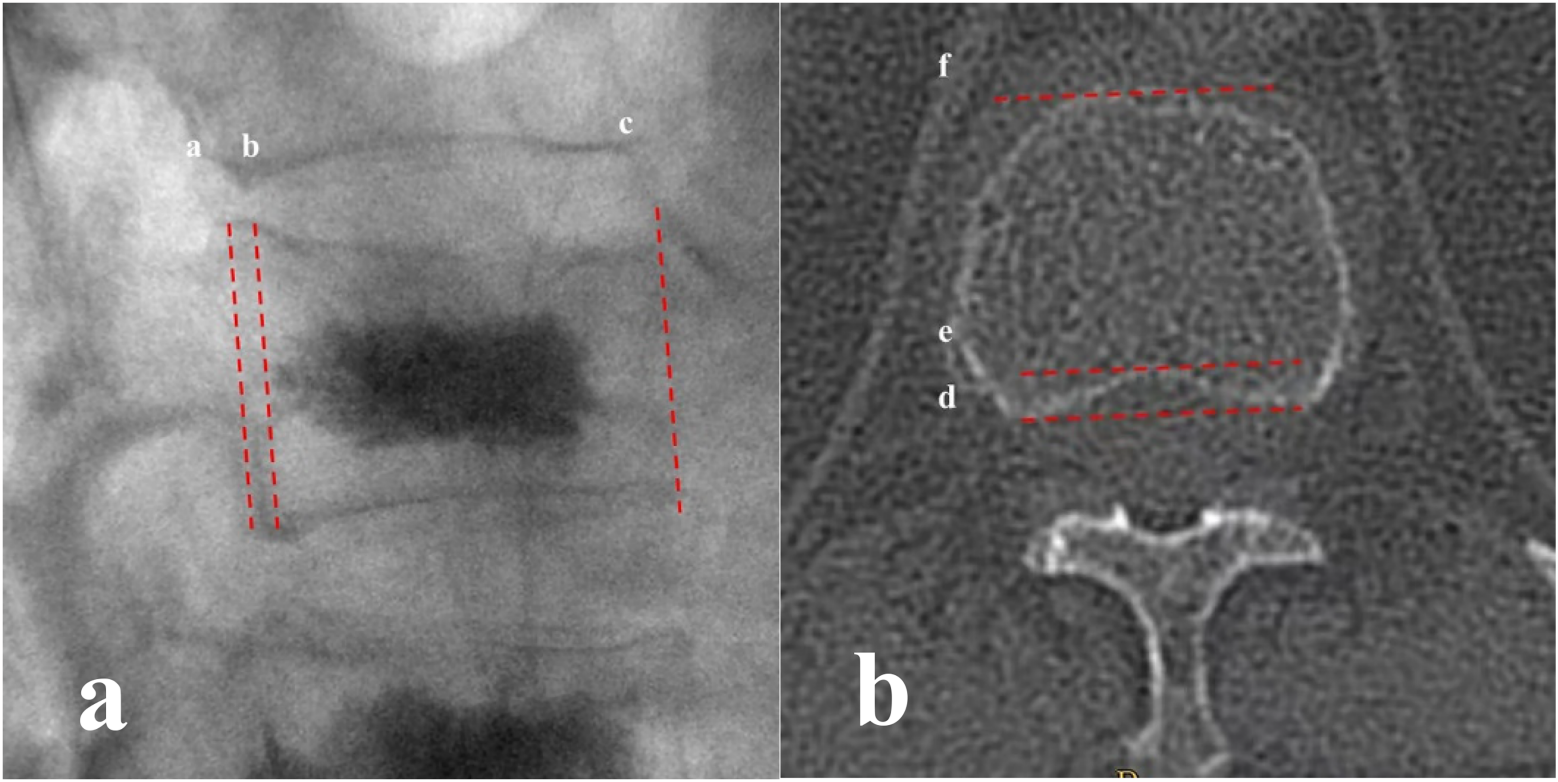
(a) In the intraoperative x-ray lateral fluoroscopy image, line a represents the posterior vertebral edge, line b represents a parallel line through the apex of the posterior edge of the bone cement dispersion, and line c represents the anterior vertebral edge line; (b) in the reconstructed CT axial images, the cortical apexes on both sides of the posterior vertebral edge were identified and connected to form line d. Lines e and f were drawn parallel to line d through the apex of the posterior vertebral depression and the anterior vertebral edge, respectively.
